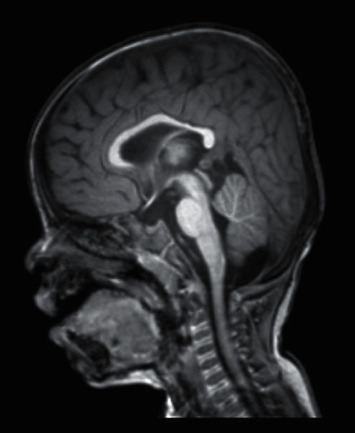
Brain MRI showing normal and progressive myelination but mild loss of volume in the corpus callosum.
